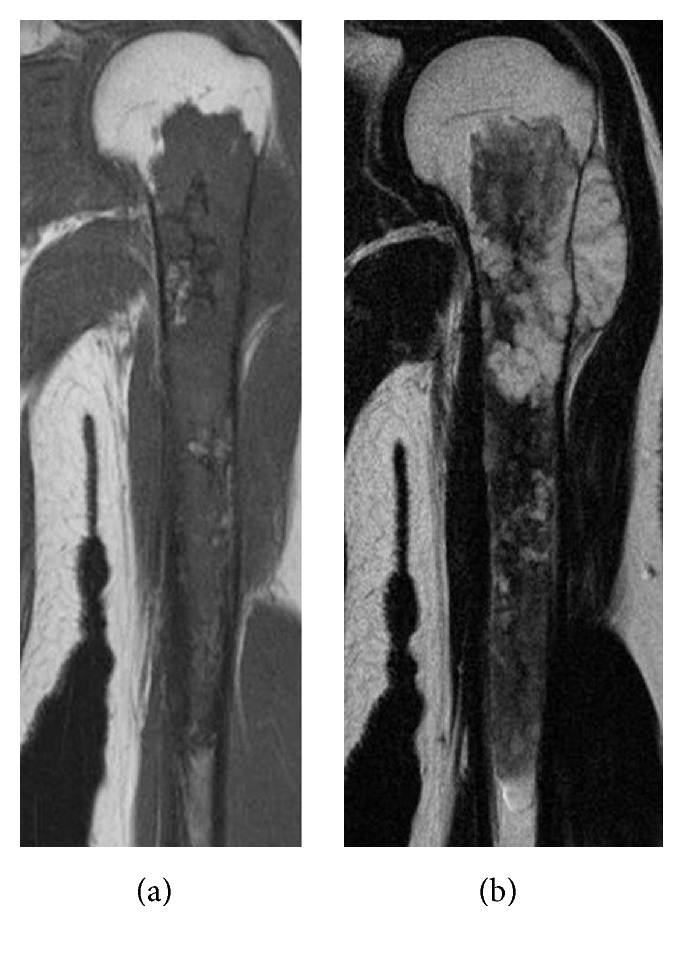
MRI findings of the left humerus. (a) T1 weighted imaging of the coronal view shows isosignals extending from the metaphysis to the diaphysis. (b) T2-weighed imaging shows intramedullary heterogenous changes with extraosseous high signals in the proximal humerus.
